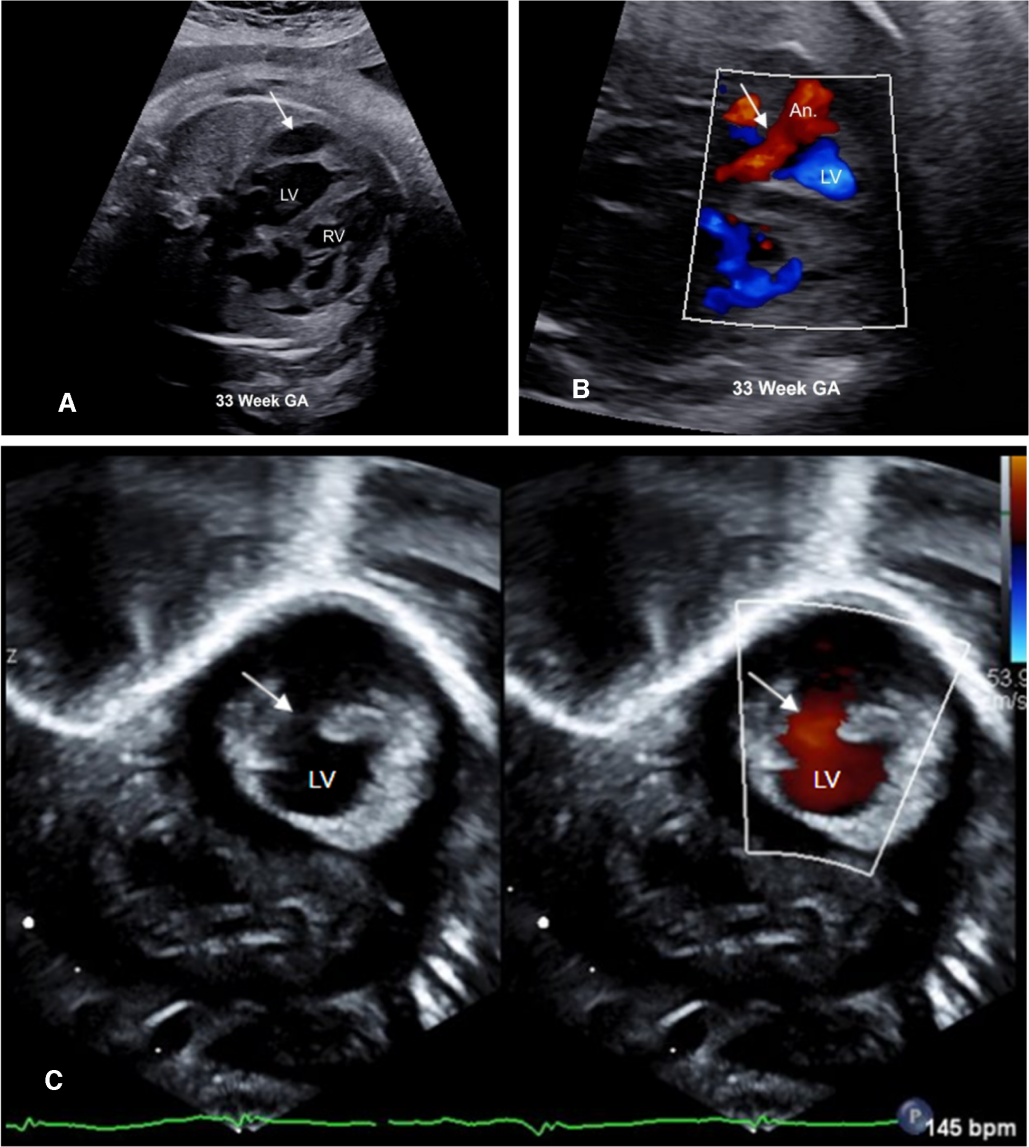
A Echocardiogram in a 33-week gestation fetus referred for evaluation of arrhythmia demonstrating hypoechoic lesion in the left ventricular wall (*arrow*). B Color Doppler image showing gap in the left ventricular wall (*arrow*) with flow into the aneurysm (*An*). C Postnatal echocardiography from a subcostal sagittal view confirmed the diagnosis of ventricular aneurysm. The LV to aneurysmal connection (*arrow*) is shown on split screen image. *LV*, left ventricle; *RV*, right ventricle; *An*, aneurysm
